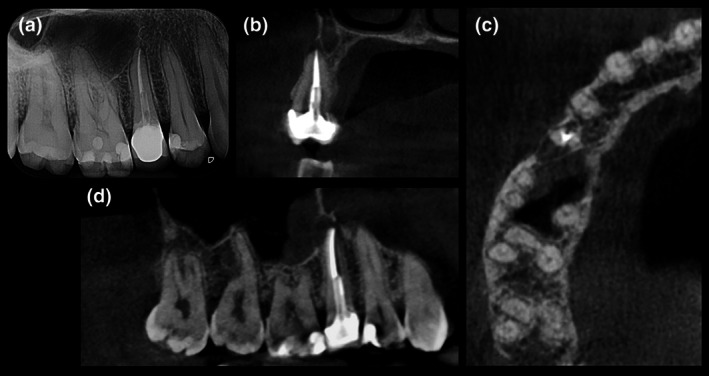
(a) Pre‐operative intraoral radiograph; (b–d) Pre‐operative CBCT, coronal, horizontal axial, sagittal view showing apical radiolucency of tooth 1.5 and inflammatory reaction of the maxillary sinus.
